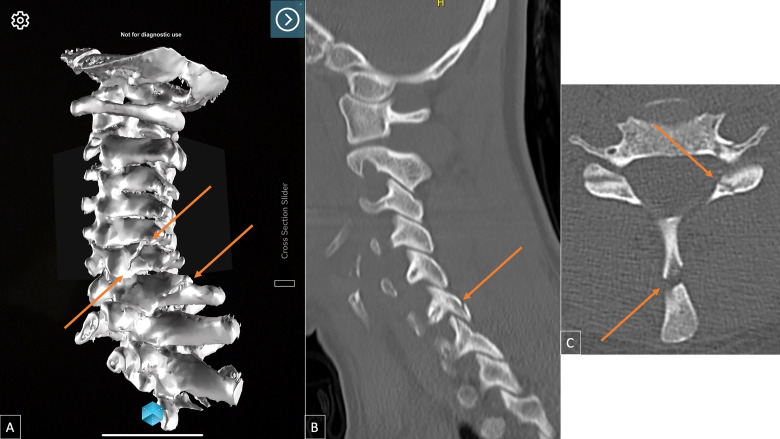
Representative images of a patient’s C6 pedicle/laminar, C6–7 facet, and C7 spinous process traumatic fractures as visualized on (A) augmented reality software, (B) sagittal computed tomography, and (C) axial computed tomography.
